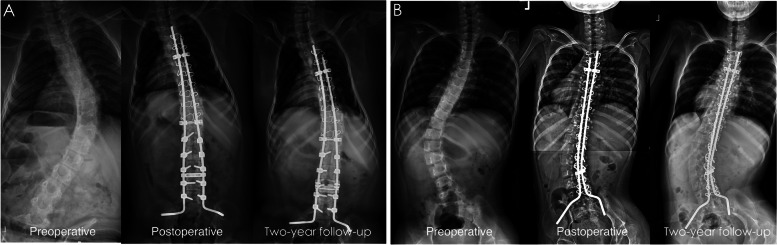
Representative preoperative, postoperative and two-year follow-up anterior-posterior radiographs of patients who received the (A) hybrid construct and (B) total sublaminar wire construct
